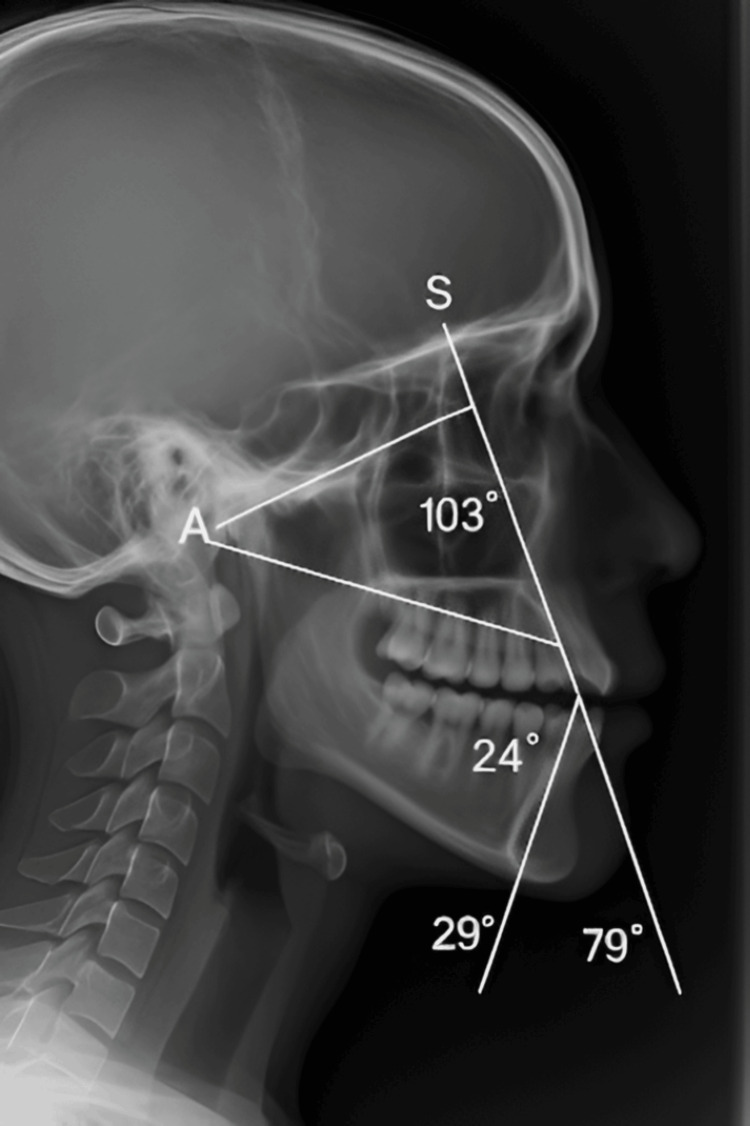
Anteroposterior cephalogram demonstrating transverse craniofacial relations and maxillomandibular alignment. Key cephalometric landmarks (S, N, ANS, PNS, Go, Me, Gn, and Pog) are indicated. Lateral cephalometric radiograph with cephalometric landmarks marked for sagittal and vertical analysis. S: Sella; N: Nasion; A: Point A (subspinale); B: Point B (supramentale)
